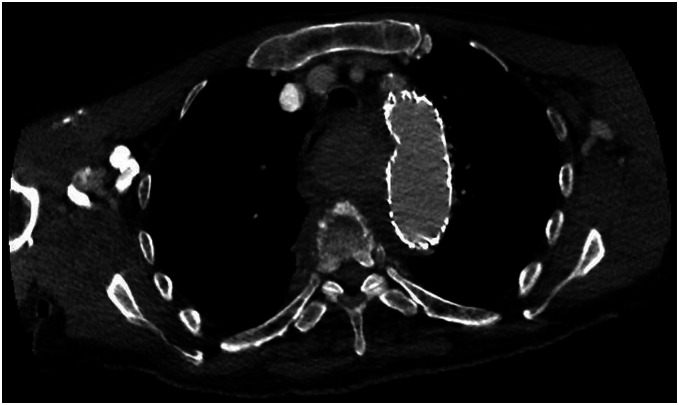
CTA scan showing progressive compression of the trachea and esophagus, stable aneurysm diameter (56 mm) and suspicion of a type 1b endoleak.
